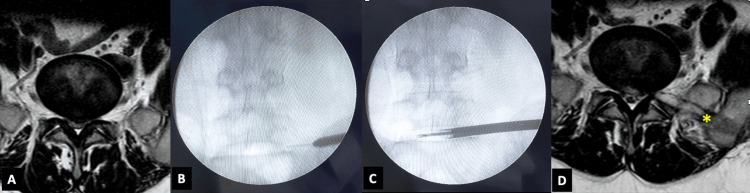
Needle insertion, access, and foraminoplasty in transIliac transforaminal endoscopic lumbar discectomy. (A) MRI of a 53-year-old male showing L5-S1 disc prolapse (Michigan State University Classification 1A), central disc prolapse. (B) The patient was operated on using transiliac transforaminal endoscopic discectomy. Iliac reaming is shown to 9 mm. Alar reaming was also needed. (C) The working cannula and endoscope are placed near the horizontal, para-centrally, with the reach of straight instruments, going to the opposite side. (D)  Immediate postoperative MRI shows adequate decompression. The co-planner iliac and alar reaming track is visible (yellow asterisk). The patient experienced significant symptom relief.
